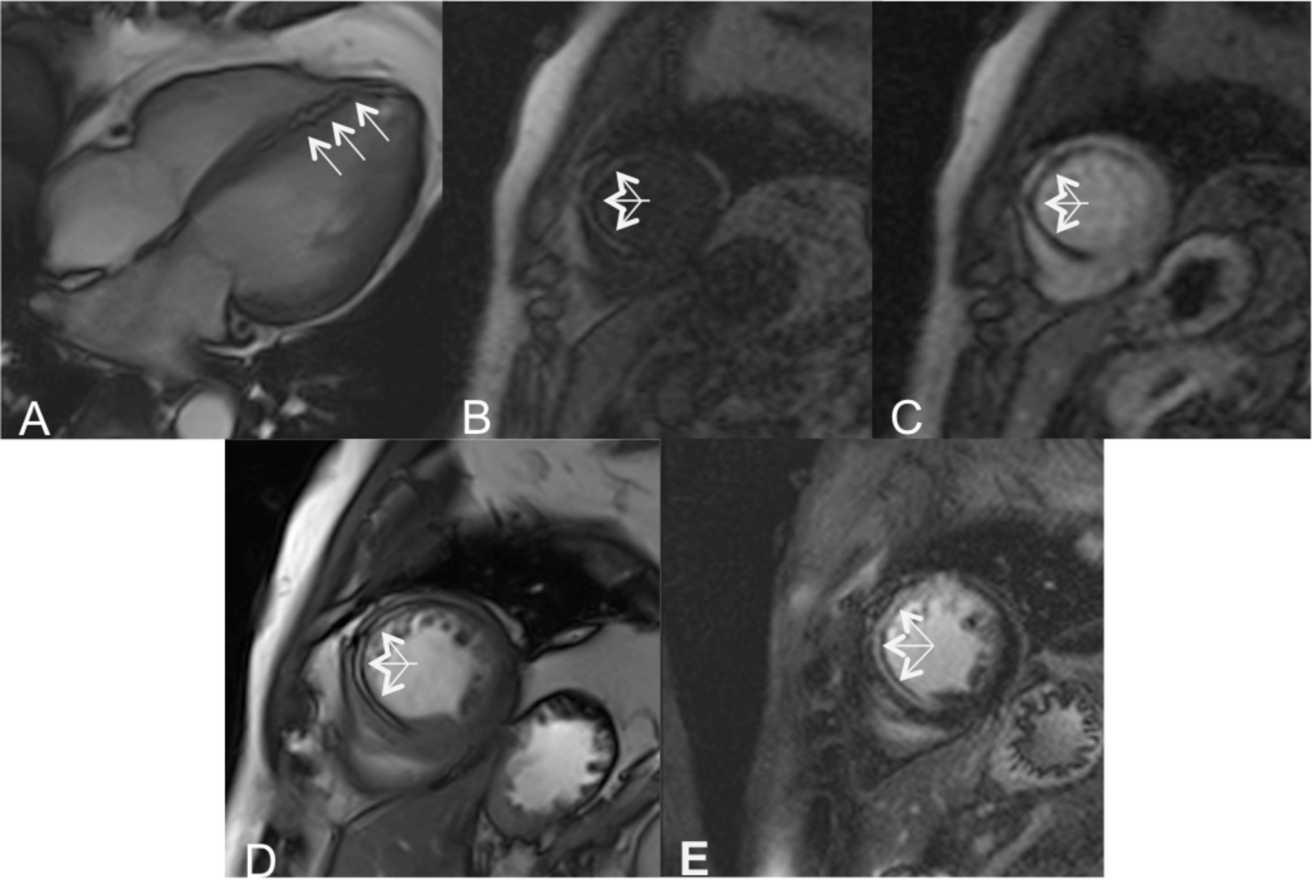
Imaging of lipomatous metaplasia A linear high signal lesion with peripheral low signal ‘etched’ appearance due to chemical shift artifact (arrows) in four-chamber and short-axis balanced SSFP in the mid anteroseptum consistent with lipomatous metaplasia (A, D). T1-weighted short-axis gradient-echo image demonstrates high signal intensity (arrows) in the mid-anterior-septal segment (B). A severe first-pass perfusion defect is seen (arrows) in the corresponding myocardial segments following the administration of gadolinium (C). The late gadolinium enhancement (LGE)-balanced SSFP image shows a high signal, which is due to fatty metaplasia rather than fibrosis (E) SSFP: steady-state free precession
